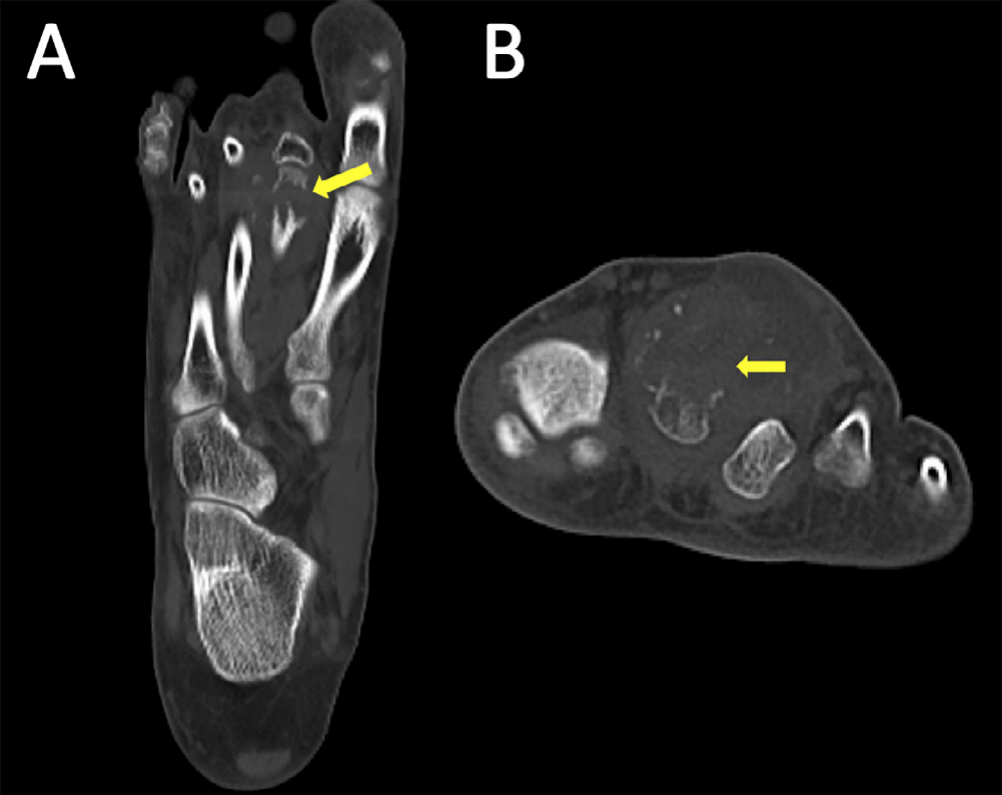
Long axis (A) and short axis (B) Cone beam CT images of the left foot. There is an expansile soft tissue lesion centered on the second metatarsal neck (arrows). There are several foci of calcification within the lesion, similar in appearance to a chondroid matrix.
